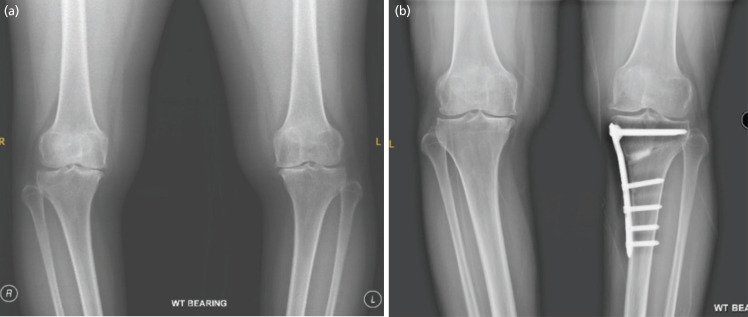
(a) Pre-operative and (b) one-year post-operative left MOWHTO AP Knee radiograph showing a united osteotomy and opening up of medial joint space.
